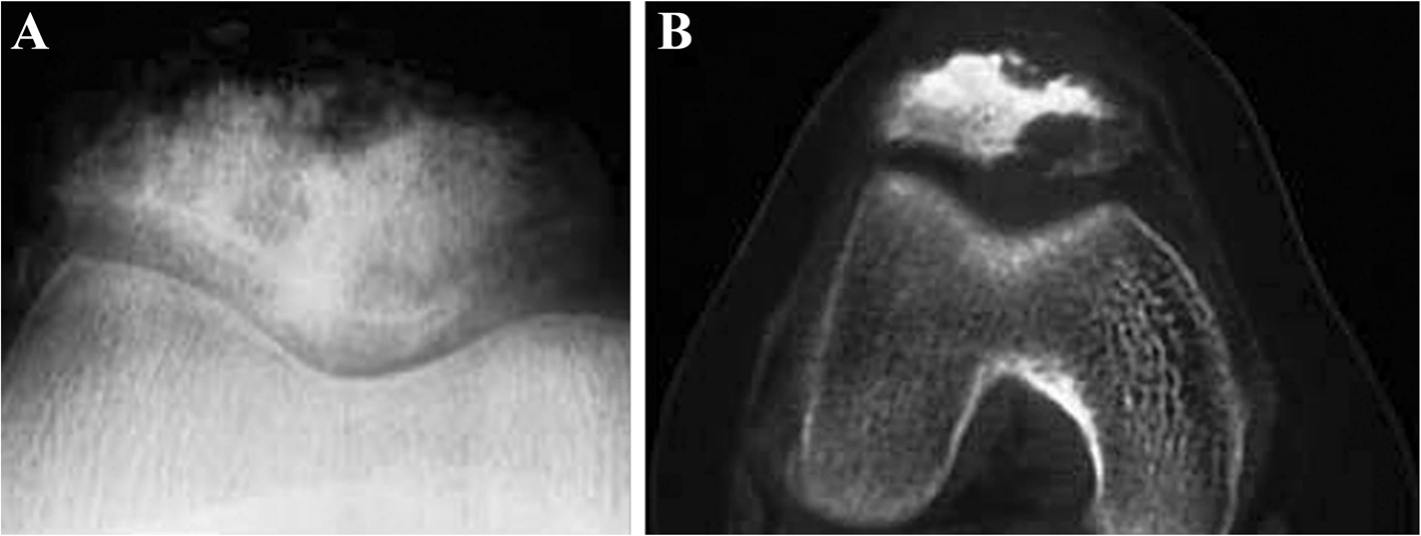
Patellar leiomyosarcoma. (A) Plain radiograph shows a mixed lytic and sclerotic lesion of patella. The margin of the lesion is ill defined and associated with cortical breach. (B) CT scan reveals multiple lytic lesions of the patella with a sclerotic rim and cortical disruption.
